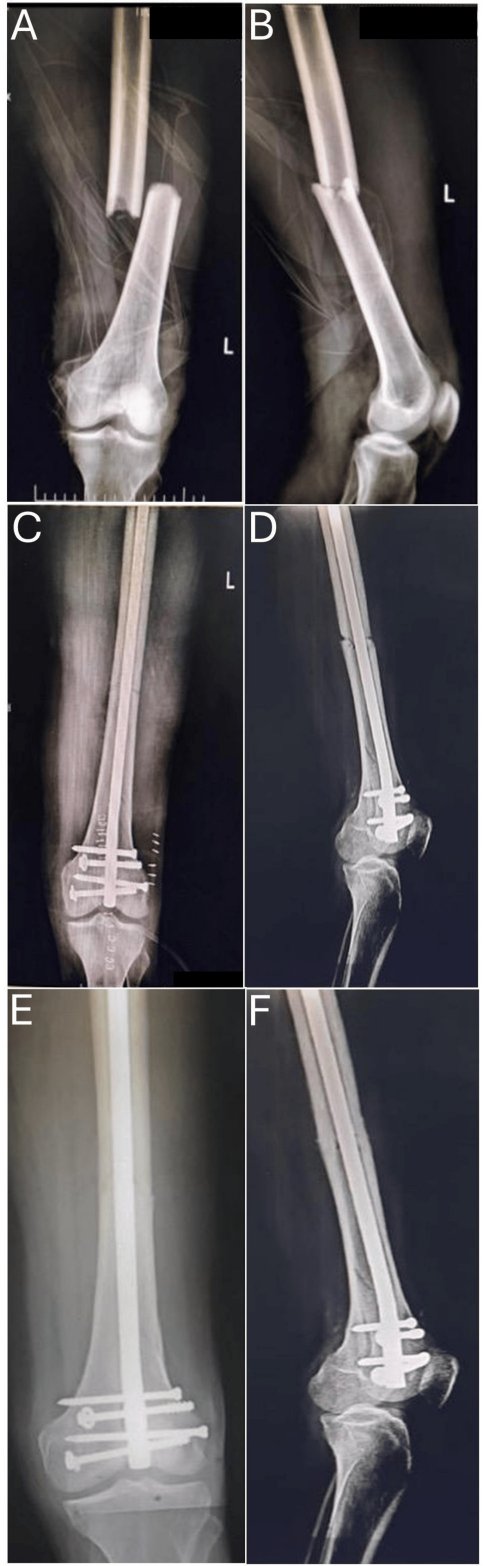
Multifunctional retrograde femoral nail fixation - image 1 (A) Preoperative anteroposterior radiograph of distal femur fracture. (B) Preoperative lateral radiograph of distal femur fracture. (C) Postoperative (immediate) anteroposterior radiograph. (D) Postoperative (immediate) lateral radiograph. (E) Postoperative (one-year follow-up) anteroposterior radiograph. (D) Postoperative (one-year follow-up) lateral radiograph
